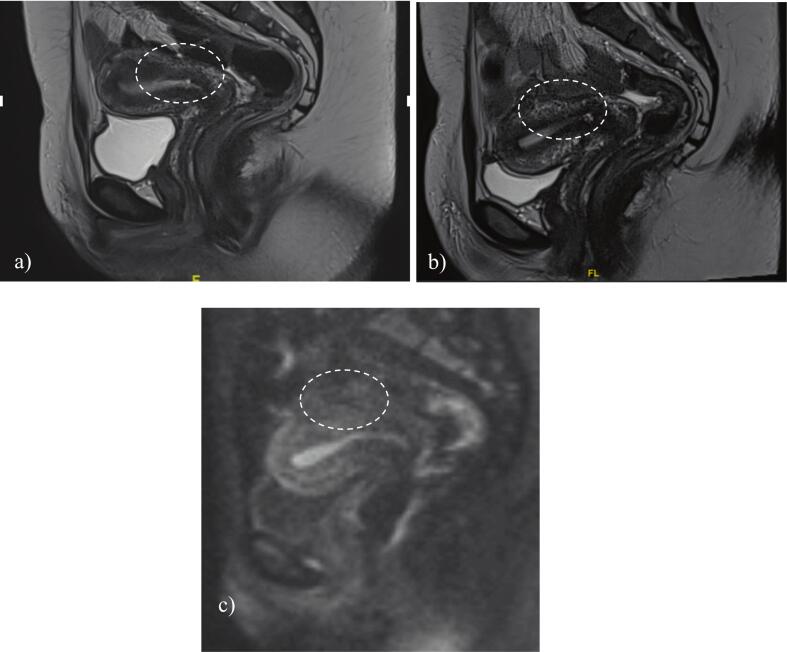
a) pelvic mri two months after treatment with pembrolizumab; b,c) Pelvic MRI 13 months after treatment with pembrolizumab.
